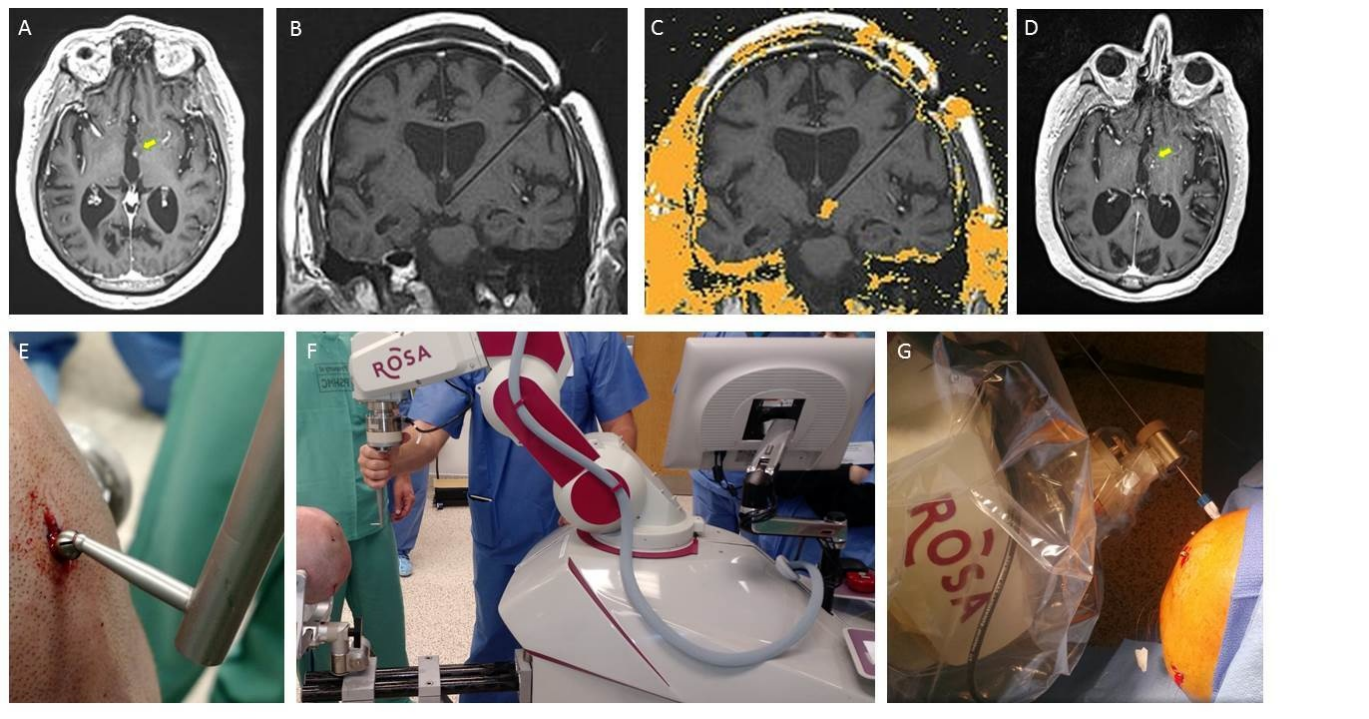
Imaging and operative details for a robot-assisted, frameless stereotactic laser ablation for an adult hypothalamic hamartoma A: Axial T1 post-contrast MRI demonstrating the left HH (yellow arrow); B: Coronal T1 MRI demonstrating accurate stereotactic placement of Visualase catheter; C: MRI thermography derived area of predicted lesion; D: Axial T1-post-contrast image on postoperative-day 1 demonstrating the lesion confined to the HH (yellow arrow); E: Demonstration of the ROSA registration arm interfacing with the Medtronic bone fiducial; F: Operative positioning of the ROSA robot and patient for registration and eventual Visualase catheter placement; G: Photograph demonstrating placement of the Visualase skull bolt with the ROSA robot in poly-axial guidance mode.
